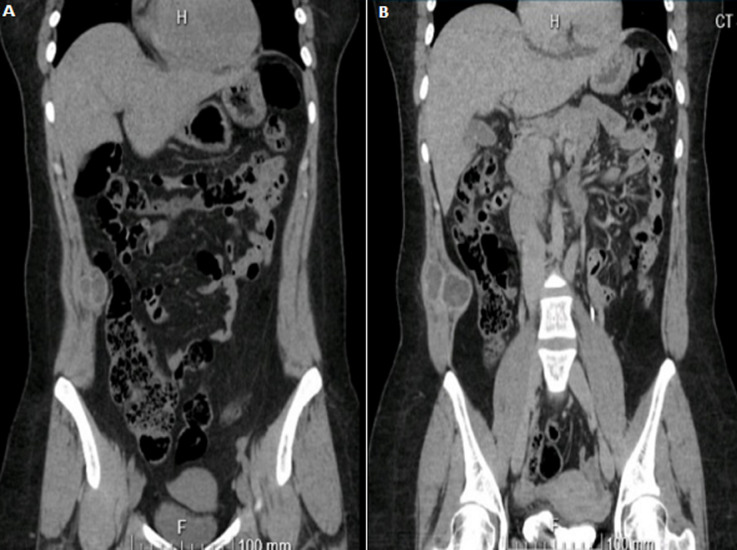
CT abdomen showing venous phase (A), arterial phase (B) a complex abdominal wall mass
